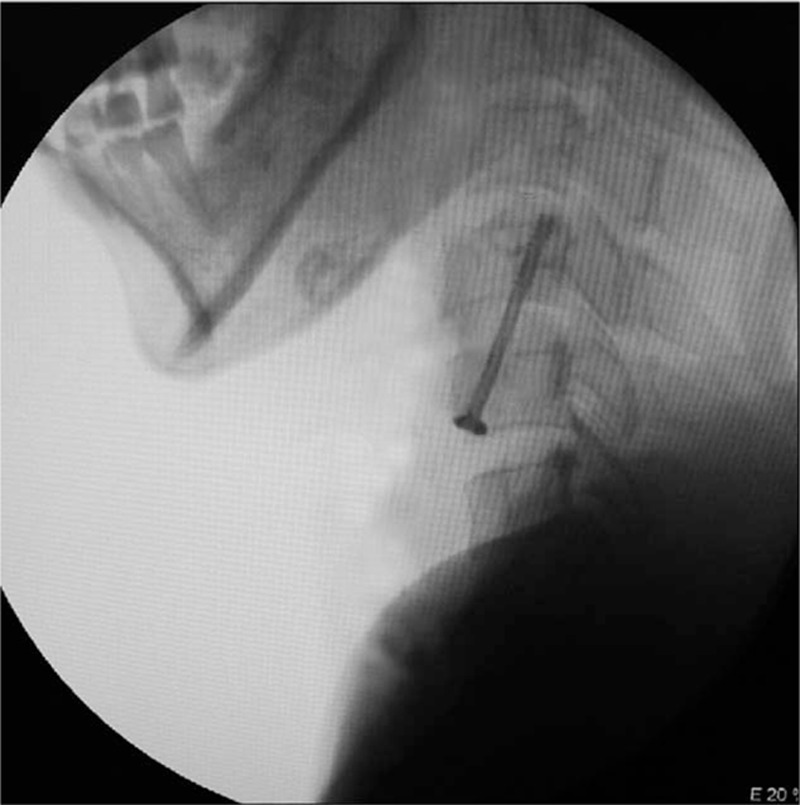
X-ray image of ATAS fixation. A partially threaded self-tapping cannulated screw with an upper oblique angle, passing through the lower vertebral body and the intervertebral space into the upper adjacent vertebral body. ATAS = anterior transdiscal axial screw.
